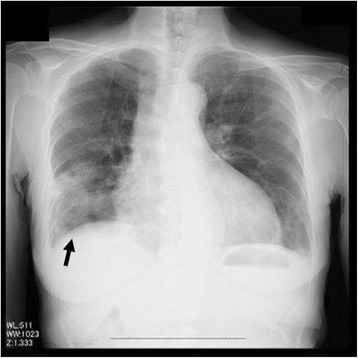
Chest x-ray showing enlarged heart associated with infiltration of both lung fields, particularly in the lower right area (*black arrow*)
